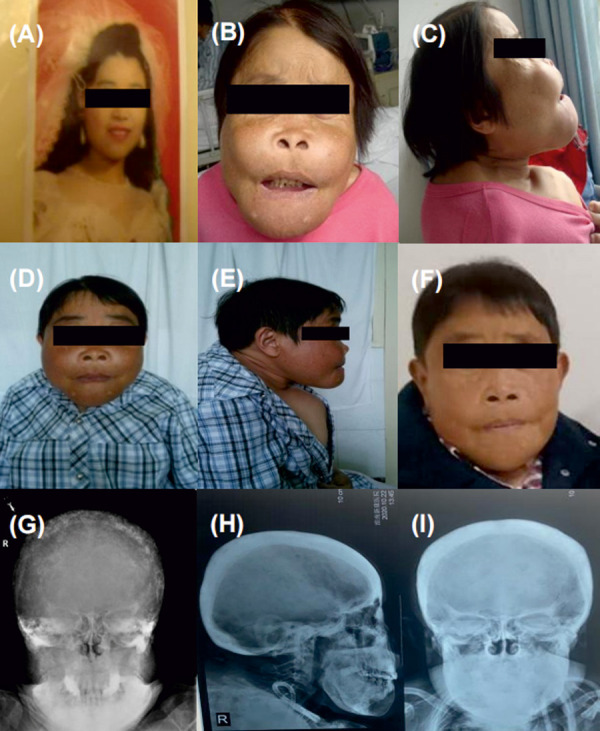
Comparison of facial deformity and skull “salt and pepper” appearance in patient with Sagliker syndrome before and after surgery. A: The patient’s normal facial appearance at the age of 20. B, C: Lion-like facial appearance before parathyroidectomy, characterized by craniofacial deformities, inability to fully close the lips, dental abnormalities, severe thoracic and spinal deformities, and a reduction in height from 162 cm to 129 cm. D, E: One year after parathyroidectomy, craniofacial deformity improved and height increased to 133 cm. F: Eight years after parathyroidectomy, craniofacial deformity improved even more significantly. G: Pre-parathyroidectomy frontal skull X-ray showed cranial thickening, accompanied by sclerosis and lytic changes, increased bone density, and bone destruction with “salt and pepper” appearance. H, I: Significant relief in skull X-rays observed at 8 years post-parathyroidectomy.
